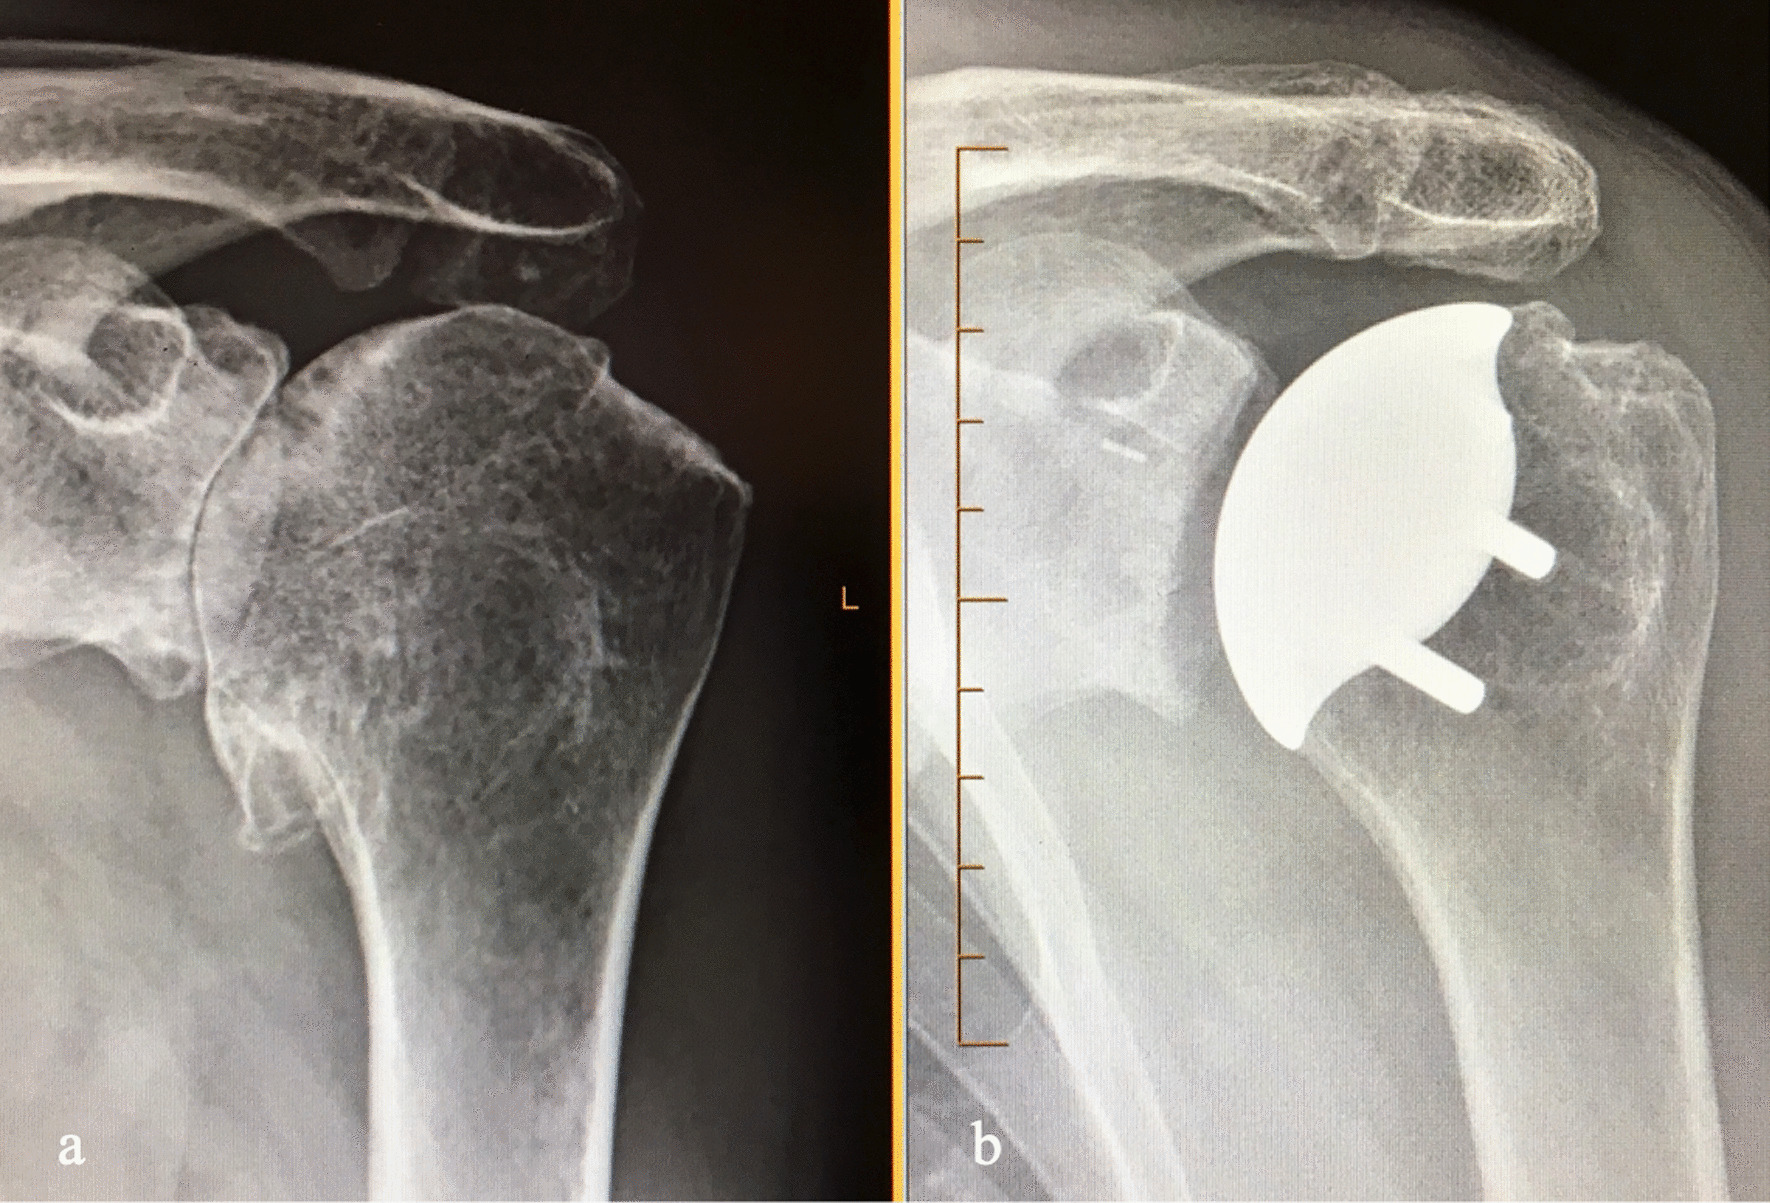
a Pre- and b Postoperative true AP radiographs with no evidence of missed inferior humeral neck osteophytes
